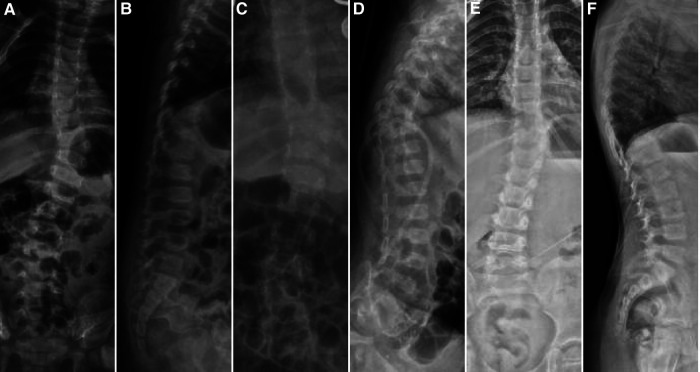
Case 14, female, 9 months old. Preoperative orthogonal (A) and lateral (B) x-rays showing the left hemivertebrae at T10. Intraoperative hemivertebra resection was performed *via* the posterior approach, and postoperative orthogonal (C) and lateral (D) x-rays are shown. At 3 years of follow-up, the orthogonal (E) and lateral (F) x-rays showed good correction of the coronal and sagittal deformity.
